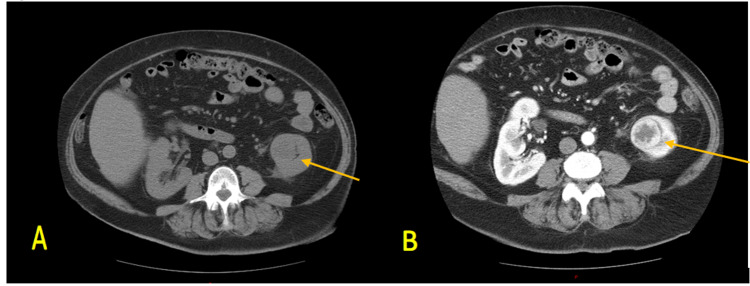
CT scan of the abdomen and pelvis without (A) and with (B) IV contrast with urogram demonstrating a 3.8-cm heterogeneously enhancing lesion of the left lower renal pole as designated by the arrow. CT, computed tomography
